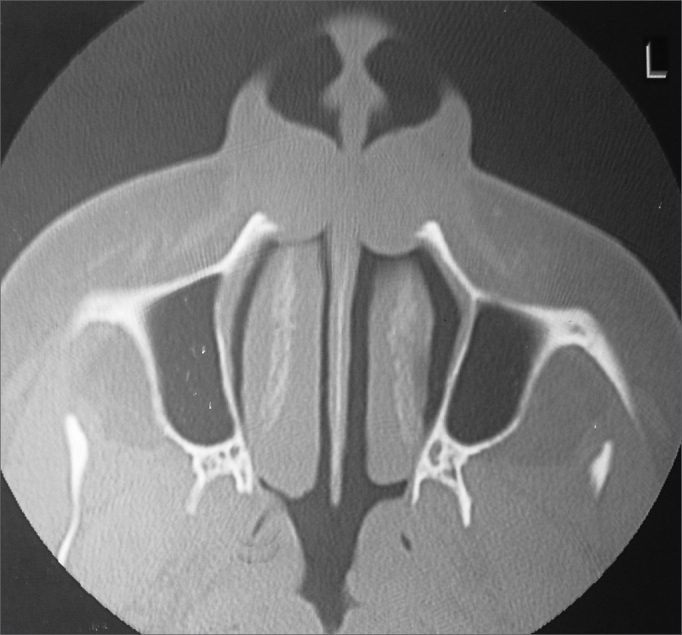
Radiological findings (computed tomography) of a nasolabial cyst – axial section of bilateral cysts.
